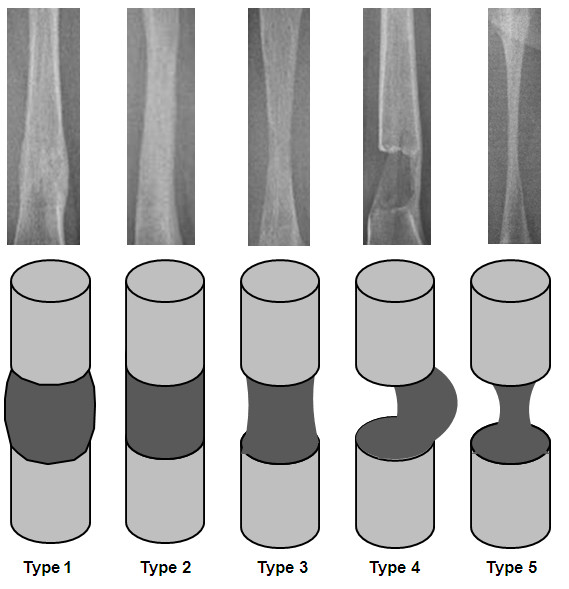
Radiographic appearance of the five types of callus shape in the Li classification [[12]: 1, fusiform; 2, cylindrical; 3, concave hourglass shape; 4, incomplete, only lateral; and 5, only central, filiform.
